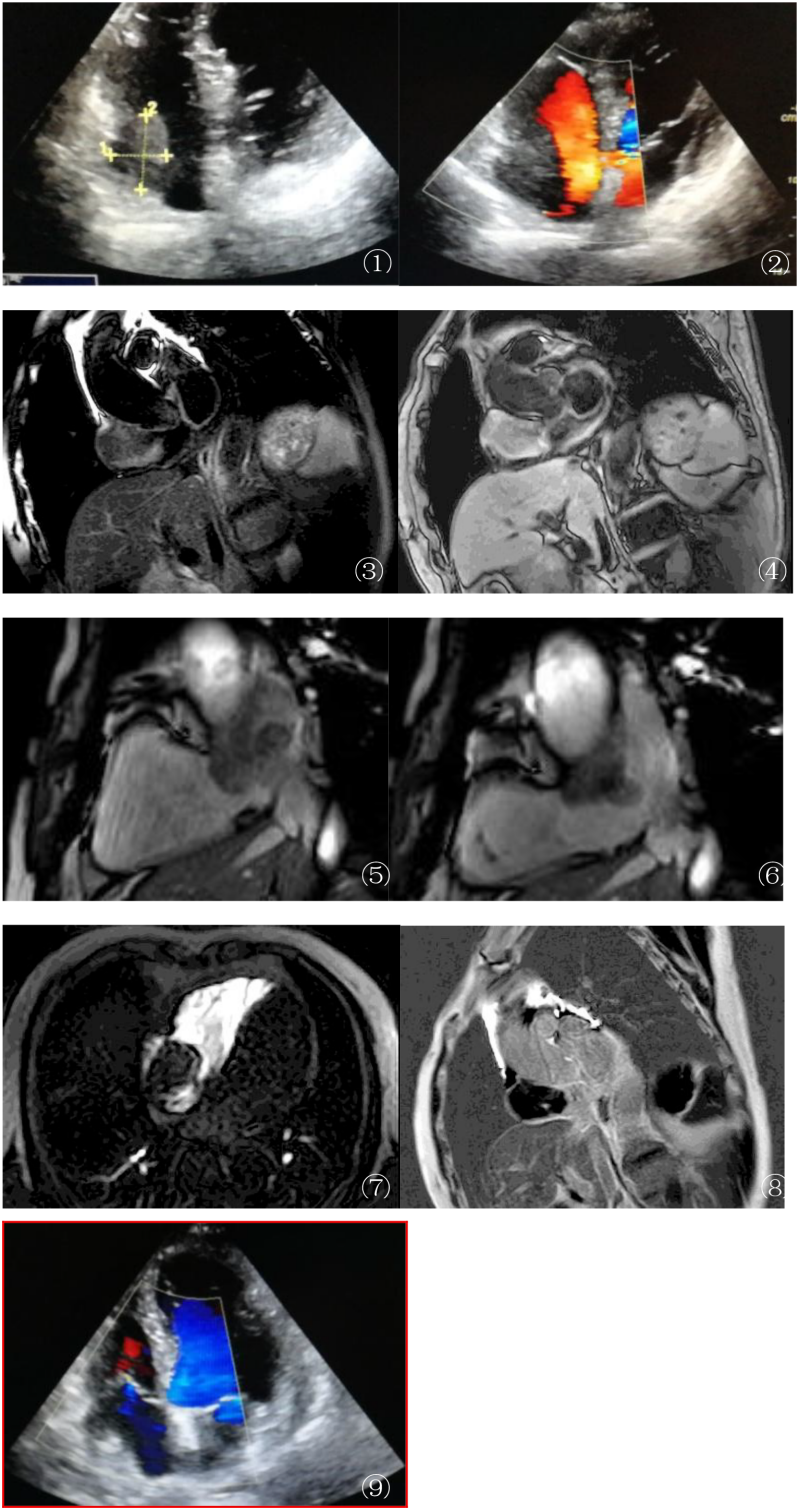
(A, B) Echocardiography findings: Transthoracic echocardiography (A) detected a 4.5-cm × 5-cm mass in the right atrium (RA). Color Doppler ultrasound (B) revealed no intralesional vascular flow, raising suspicion of either a thrombus or an avascular tumor. These initial findings lacked specificity for definitive diagnosis, highlighting the need for further imaging. (C, D) MRI anatomical imaging: Axial T2-weighted single-shot turbo spin echo (T2W–SSH–TSE) (C) and T1-weighted turbo spin echo (T1W–TSE–SA) (D) sequences demonstrated an isointense mass in the RA with broad-based attachment to the anterior and posterior walls. Adjacent structural changes were notable: the RA wall exhibited thickening and edema, and the inferior vena cava (IVC) inlet showed stenosis, indicating potential local invasion. (E, F) Cardiovascular magnetic resonance (CMR) cine sequence (balanced turbo field echo, BTFE–BH–LA): During atrial systole (E) (*left panel*), the mass protruded into the tricuspid orifice, causing secondary tricuspid regurgitation. In diastole (F) (*right panel*), the mass retracted back into the RA, closely adjacent to the superior vena cava (SVC) and IVC orifices. This dynamic motion pattern provided insights into the mechanical impact of the mass on cardiac hemodynamics. (G) First-pass perfusion MRI: Unusually, the mass showed no enhancement during the arterial phase of first-pass perfusion (F), contrasting with the typical hypervascularity observed in diffuse large B-cell lymphoma (DLBCL). This atypical feature suggested unique tumor microvascular characteristics or potential sampling bias, warranting further investigation. (H) Late gadolinium enhancement (LGE) MRI: Axial images revealed patchy, irregular enhancement of the mass, with non-enhancing central foci consistent with tumor necrosis. Notably, the edematous atrial wall showed no abnormal enhancement, helping to differentiate the tumor tissue from reactive changes. (I) During the re-examination 2 months after surgery, the patient was found to have cardiac enlargement and pericardial effusion, with no recurrence in the surgical area.
